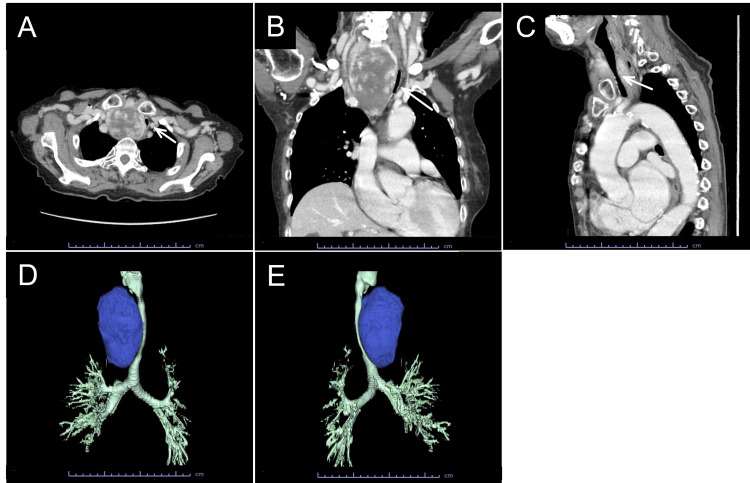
CT and three-dimensional reconstruction of a massive goiter causing severe tracheal stenosis Axial (A), coronal (B), and sagittal (C) CT images showing severe distal tracheal stenosis caused by a massive right-sided goiter. Volume-rendered (VR) anterior (D) and posterior (E) views provide three-dimensional visualization of the mass and its relationship to the trachea. White arrows indicate the critically narrowed tracheal lumen. On CT reconstruction, the stenotic segment extended from approximately 2.5 cm below the glottis to 2.5 cm above the carina, with a total length of approximately 5 cm and a minimum residual tracheal lumen of 4 × 9 mm.
